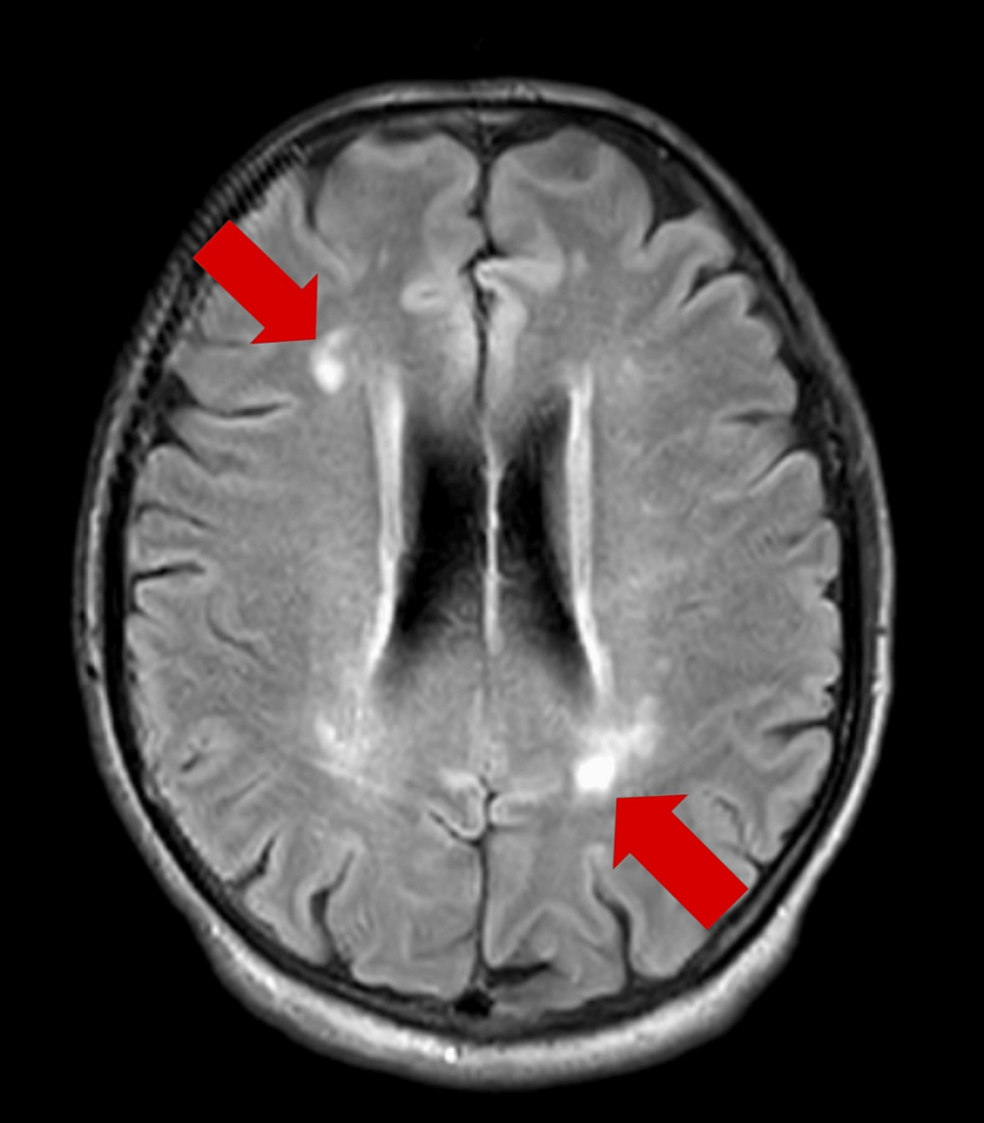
Noncontrast MRI brain image: Fluid-attenuated inversion recovery (FLAIR) axial section showing hyperintensity in bilateral subcortical and periventricular deep white matter Furthermore, there is no evidence of cortical atrophy in relation to dementia, and the patient's brain volume is good.
